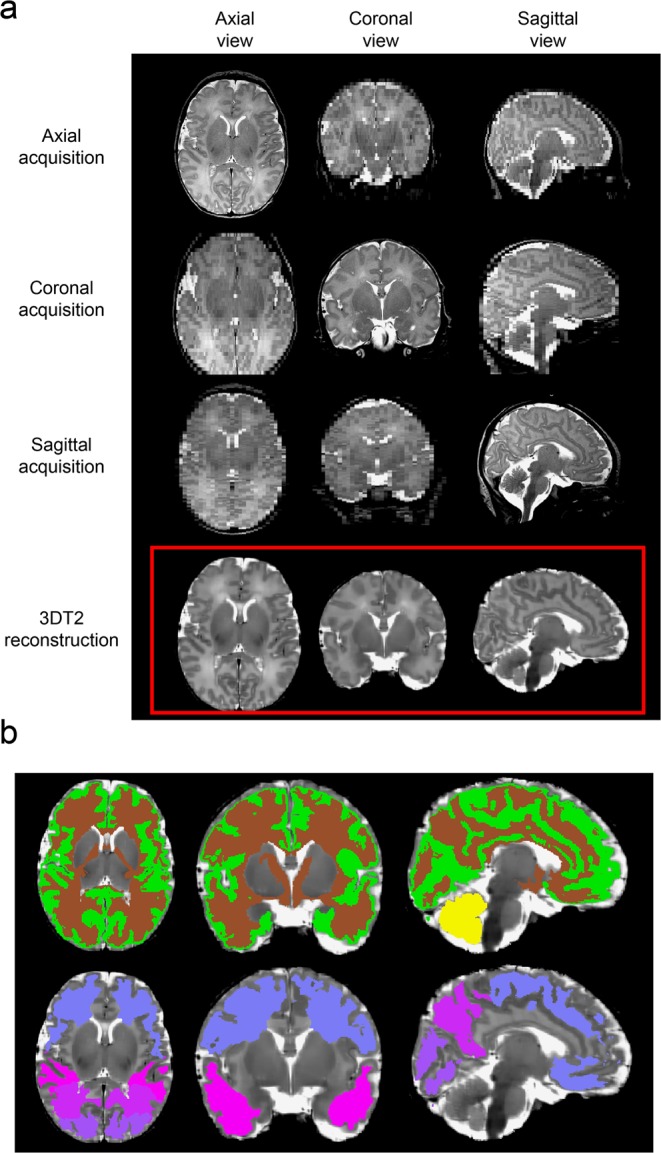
3D reconstruction and brain segmentation. (a) Reconstruction of 3D T2-weighted images (bottom row) from three orthogonally acquired images, (b) example segmentations: cortex, white matter and cerebellum (top row), and four-lobe segmentation (bottom row).
